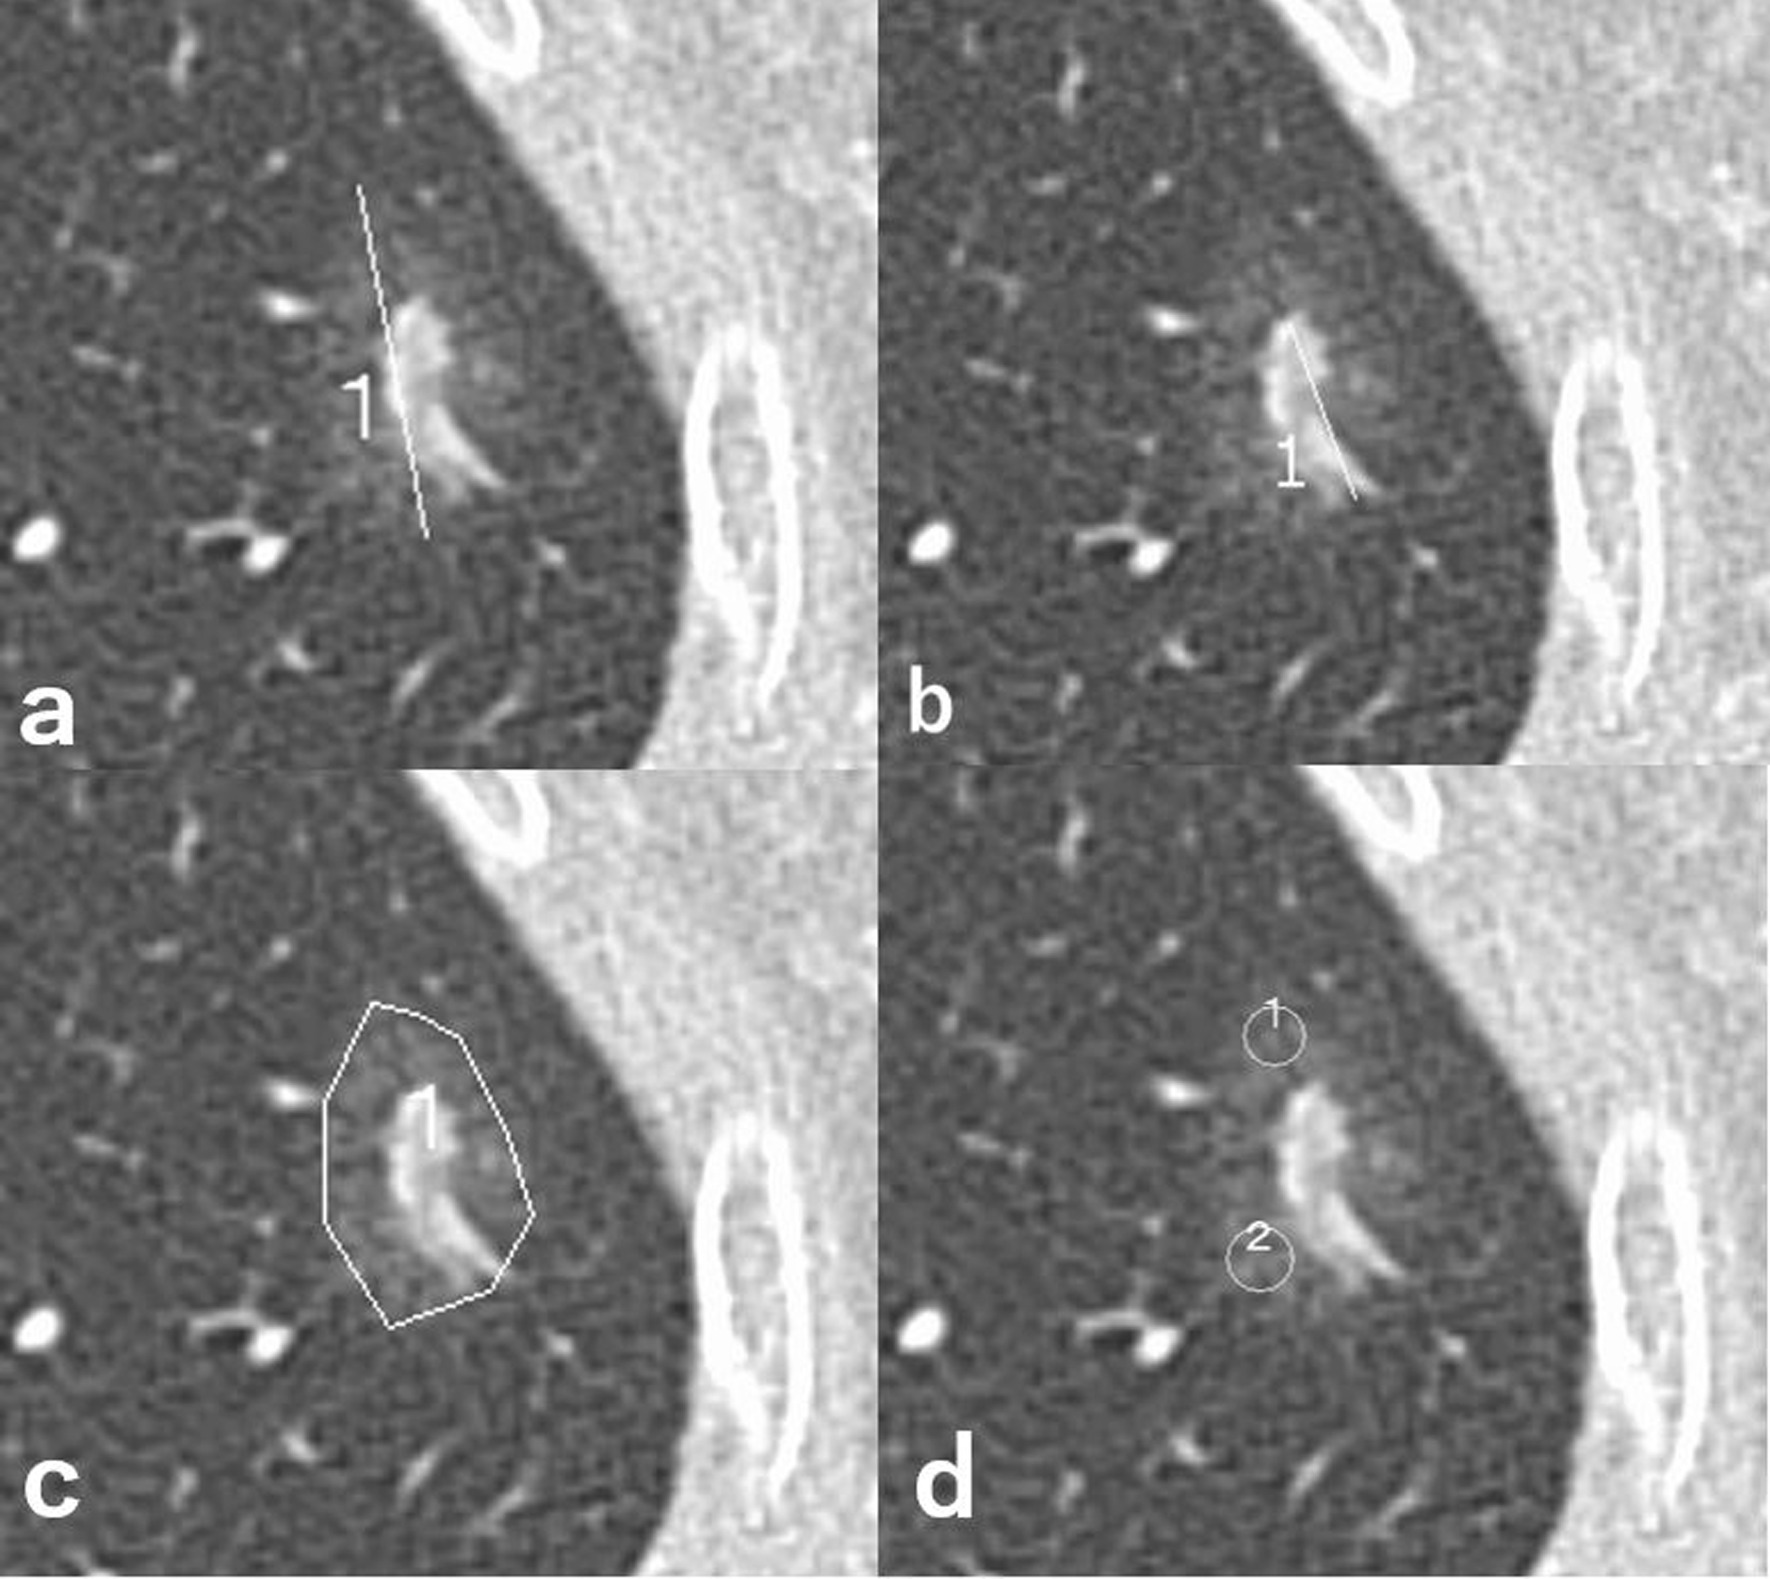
74-year-old non-smoker woman who was pathologically diagnosed with minimally invasive adenocarcinoma. Longest diameter of the entire lesion (a), longest diameter of the solid component (b), average CT value of the entire lesion (c), and average CT value of the ground-glass opacity (GGO) portion on axial slices (d)
